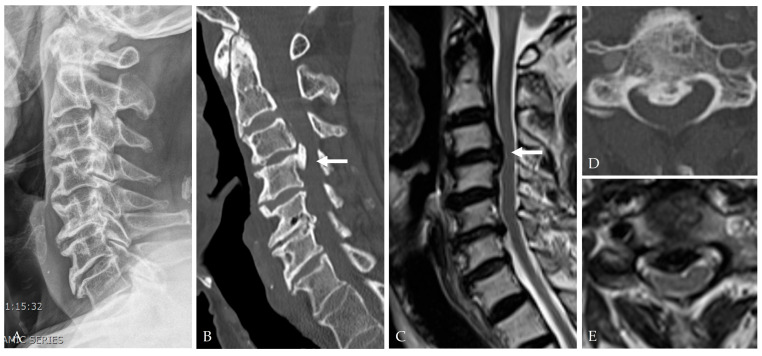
A 79-year-old male patient complained of cervical myelopathy. Latreal X-ray (A), CT (B,D), and MRI (C,E) showed C3–4 OPLL compressing the spinal cord (white arrow).
